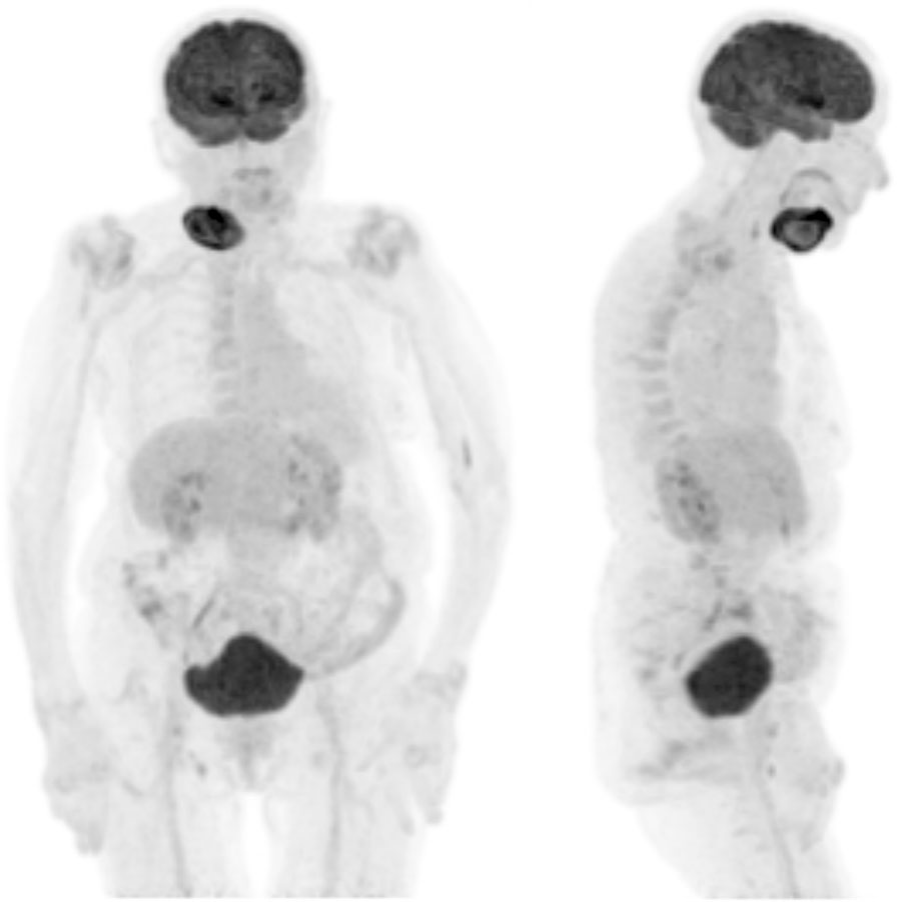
18-FDG positron emission tomography maximum intensity projection images with anterior (left) and lateral (right) views showing the avid right submandibular mass to be the only site of disease
